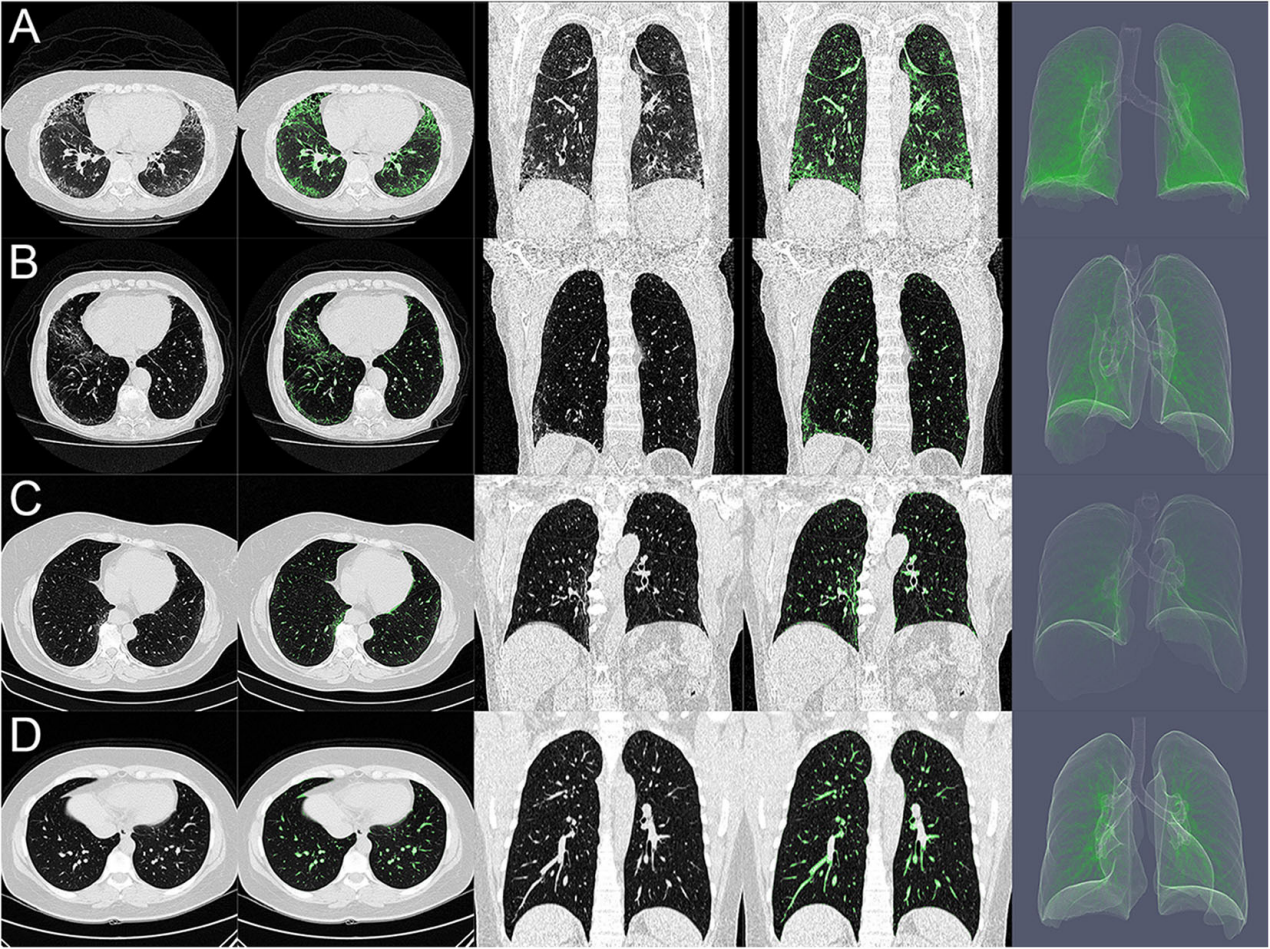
(A–D) Show axial (columns 1 and 2) and coronal (columns 3 and 4) CT scans and three-dimensional rendering (column 5) of the lung of systemic sclerosis classified as with extensive interstitial lung disease (SSc Extensive-ILD, A), as with limited interstitial lung disease (SSc Limited-ILD, B), as with no interstitial lung disease (SSc No-ILD, C), and controls (D). Note, in (A), predominance of ground glass/reticular consolidation and compressed tissue likely related to cellular/fibrotic nonspecific interstitial pneumonia at basal lung third. Those in green are represented interstitial opacities with densities ranging from −500 and +50 HU that would represent the extent of pulmonary involvement.
